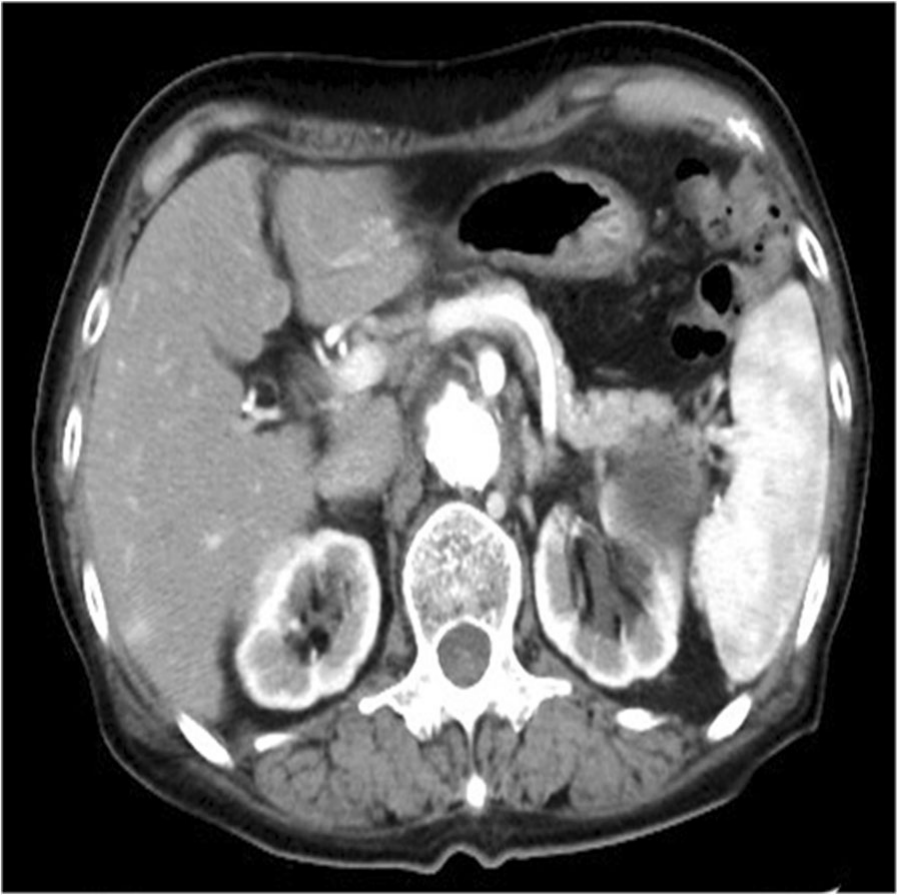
Contrast computed tomography in the arterial phase, performed on postoperative day 36. None of the findings suggested a tumor in the residual liver
